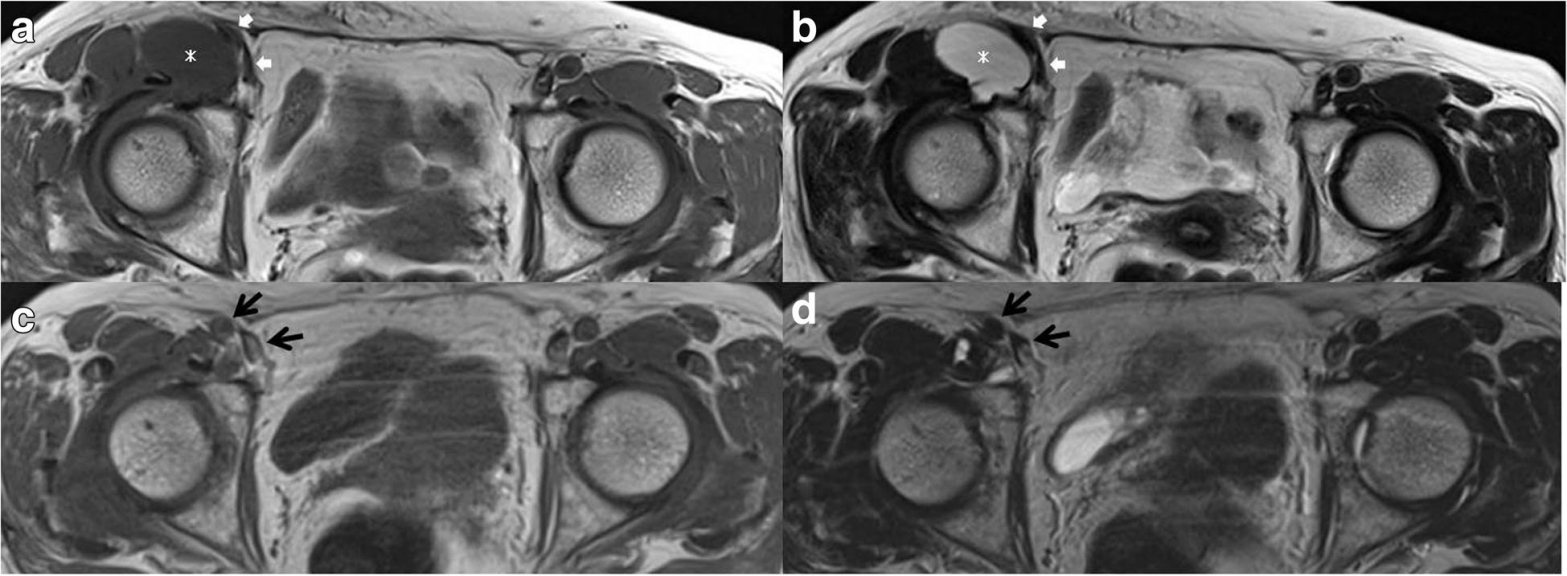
Axial T1-weighted (a) and T2-weighted (b) magnetic resonance imaging performed before needle aspiration showed that the cystic mass (asterisk) had increased in size to 45 × 25 mm. The cystic mass in the right groin had compressed femoral vessels (white arrows). Axial T1-weighted (c) and T2-weighted (d) magnetic resonance images obtained at 7.5 years after needle aspiration revealed that the cyst had considerably shrunk in size and femoral vessels had been released from the compression (black arrows)
